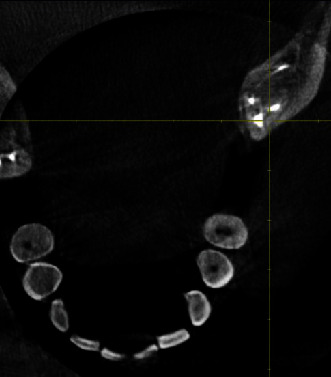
CBCT axial view (postoperative).
